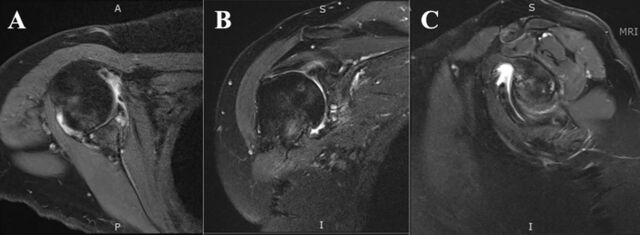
Noncontrast magnetic resonance imaging of the right shoulder in (A) axial, (B) coronal, and (C) sagittal slices of the glenohumeral joint shows degenerative changes including cartilage thinning and subchondral cystic changes of the glenoid and humeral head. Inferior labral deficiency can be appreciated on the (A) axial and (B) coronal images. Imaging also shows evidence of a high-grade articular-sided partial thickness tear of the supraspinatus tendon (A).
